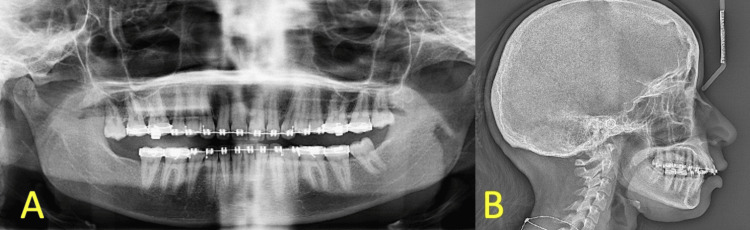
(A) Preop panoramic radiograph. (B) Preop lateral cephalometric radiograph.
